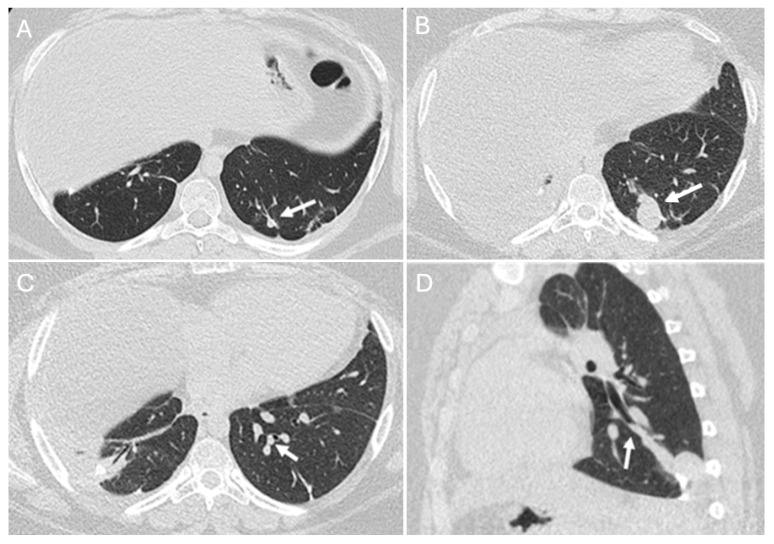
Patient with osteosarcoma and recurrent lung metastasis post metastasectomy and endobronchial metastasis. Axial non-enhanced image (A) shows a small left lower lobe nodule along the surgical line (arrow), suggesting recurrent disease. Axial non-enhanced image (B) from a follow-up CT shows an interval increase in size (arrow) representing disease recurrence. Axial (C) and sagittal (D) images from the follow-up CT show an interval development of endobronchial extension (arrows).
